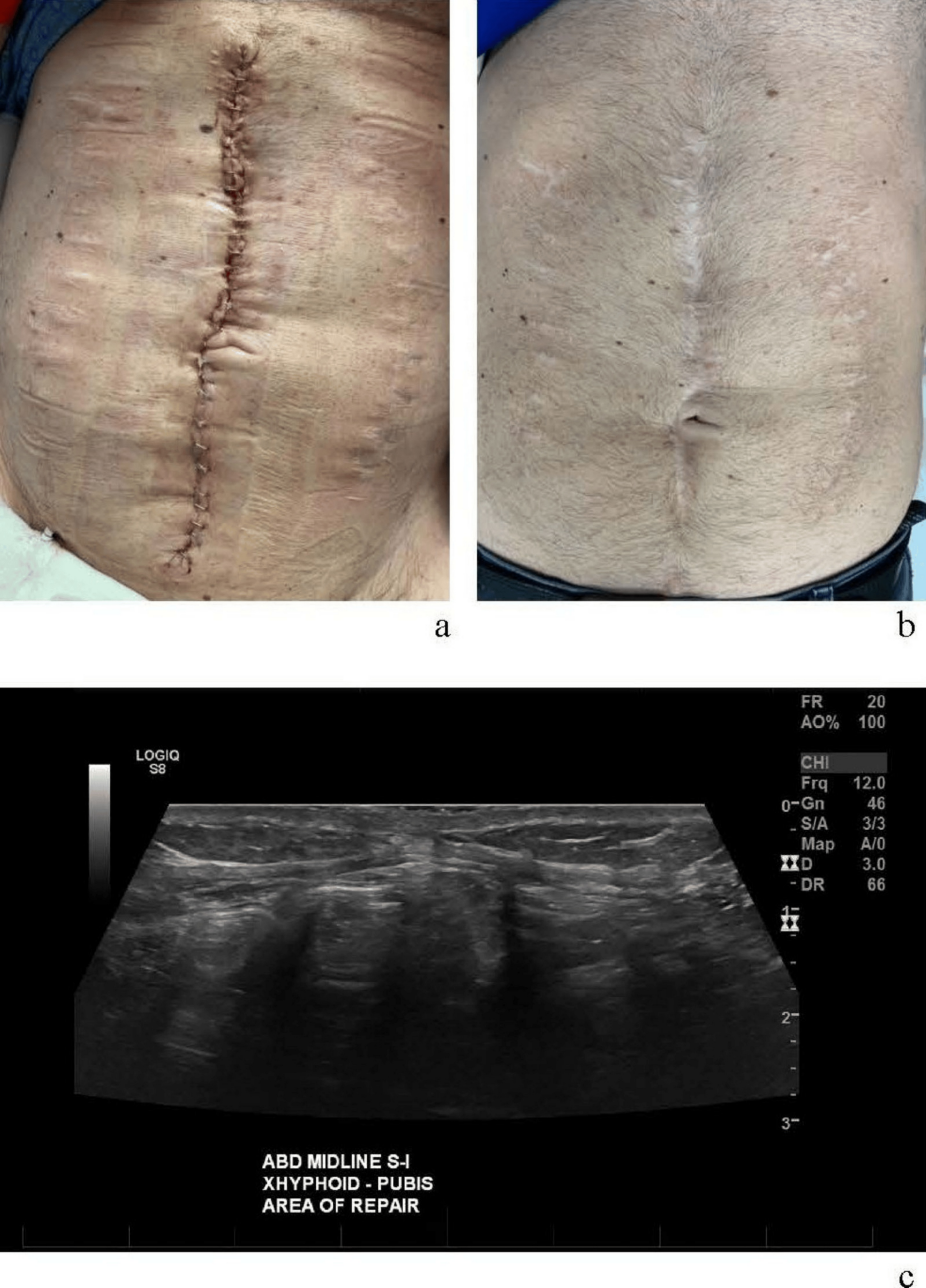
Abdominal reconstruction surgery: (a) Immediately post-op; (b) 20 months post-op; (c) 20-month post-op ultrasound, showing re-approximation of rectus muscles.
